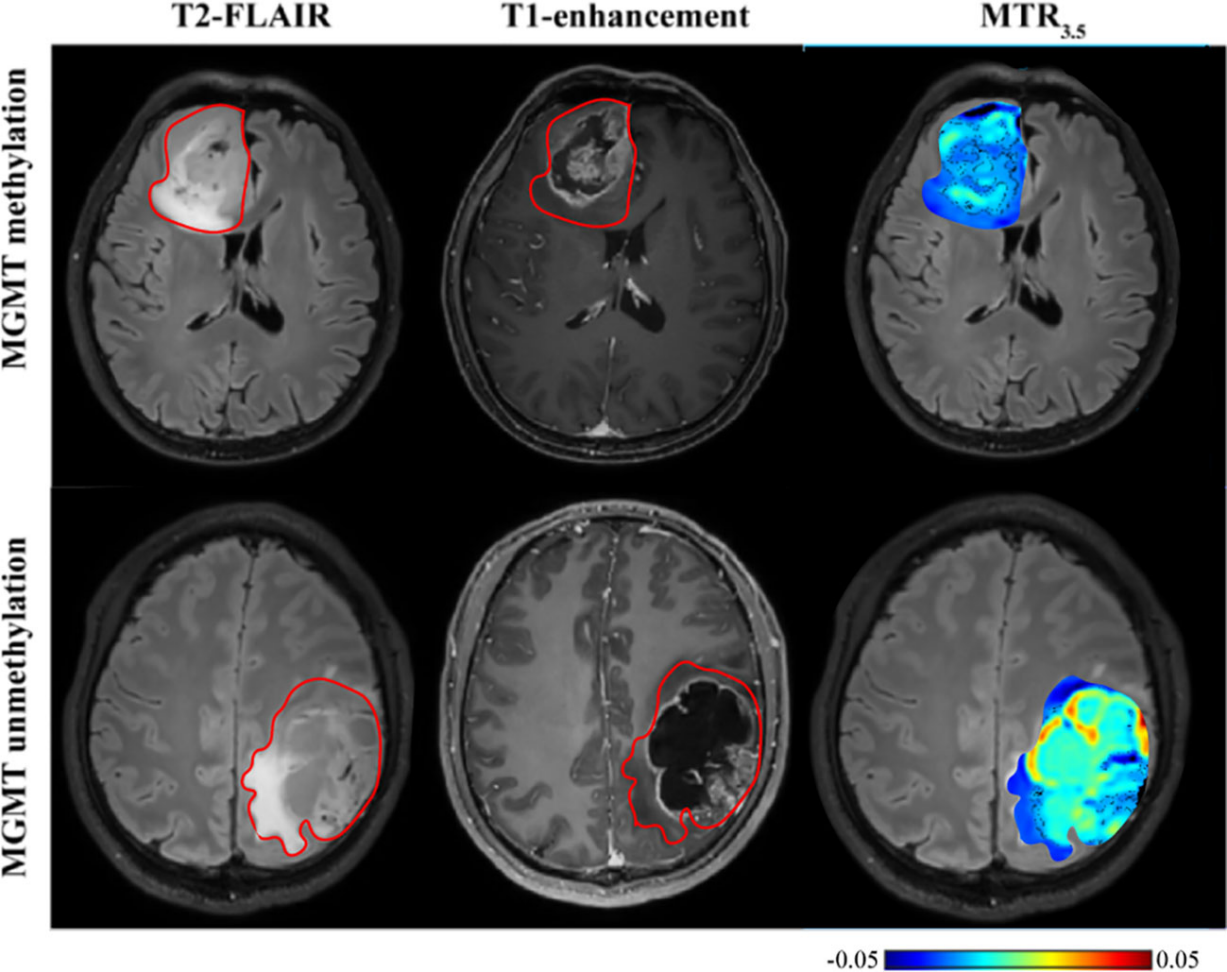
T2-FLAIR, T1-enhancement images and effective CEST derived metric map of MTR_3.5_ for differentiating between one MGMT promoter methylation glioma (53 years old female, WHO IV) and one MGMT promoter unmethylation glioma patient (30 years old male, WHO IV). MGMT promoter methylation glioma manifested higher MTR_3.5_ compared to unmethylation glioma.
